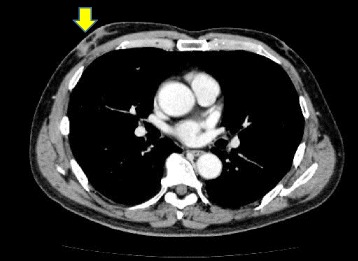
A preoperative chest CT scan showing the incisional biopsy scar (*arrow*) of the right breast carcinoma
